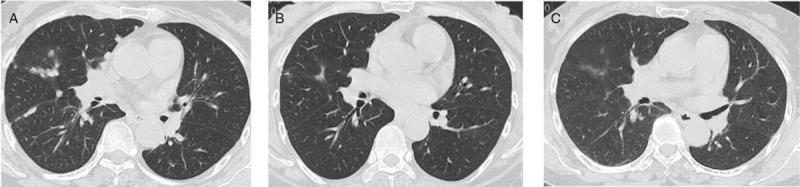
A, The first chest CT scan. B, Two cycles of chemotherapy and crizotinib. C, Six cycles of chemotherapy and crizotinib. CT = computer tomography.
